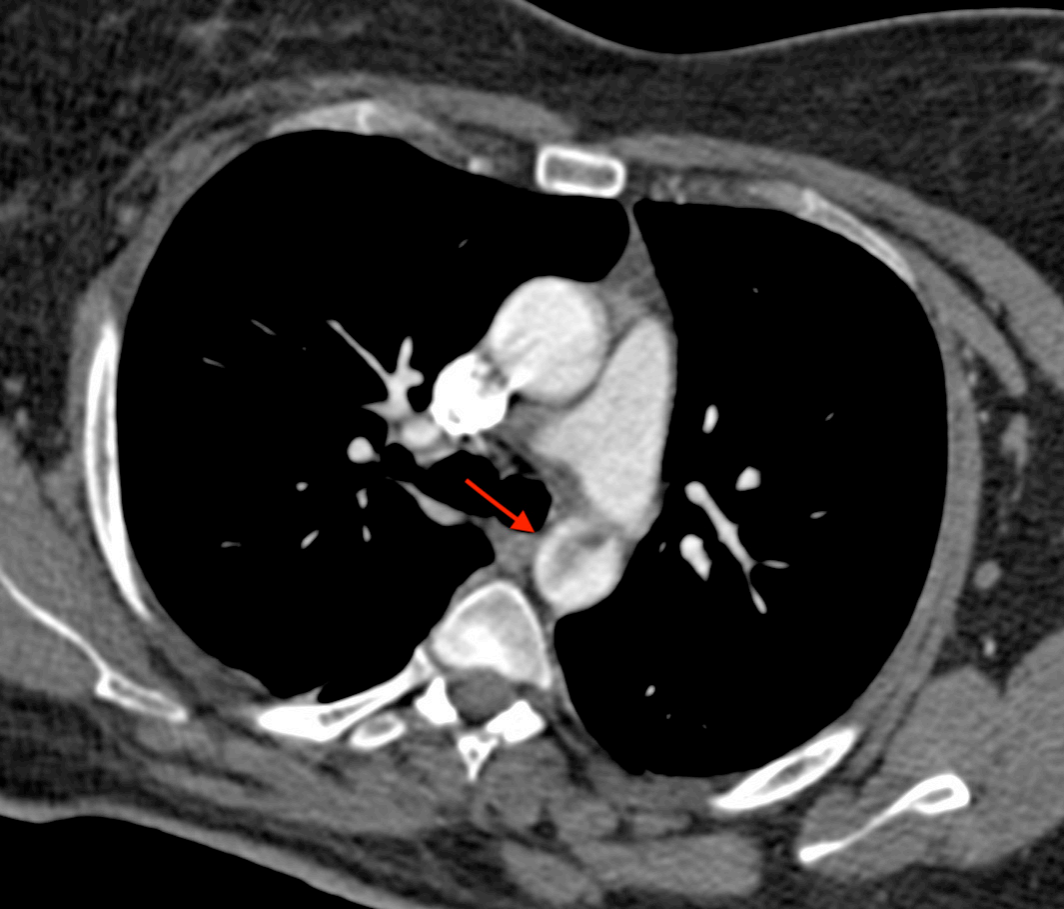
Computed tomography angiography axial scan showing the descending thoracic intraluminal aortic filling defect (red arrow).
